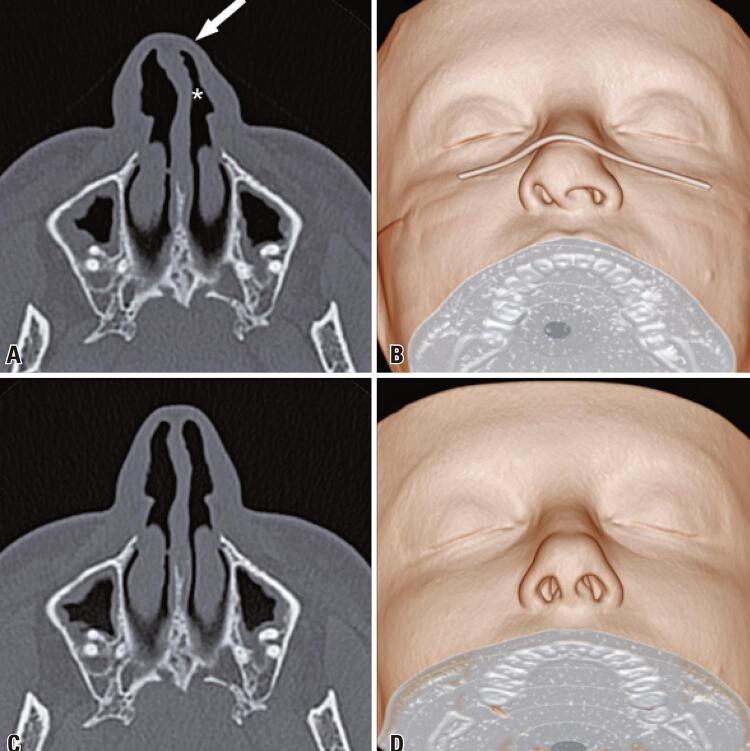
Computed tomography images of the same patient performed in 2021 (A and B) and 2019 (C and D). Axial (A) and three-dimensional volume-rendered (B) computed tomography images show significant deviation of the anterior cartilaginous portion of the nasal septum to the left, with a reduction of the left nasal vestibule (asterisk) and flattening of the nasal dorsum (arrow). These findings are associated with the use of a face mask. Axial (C) and three-dimensional volume-rendered (D) computed tomography images, performed two years before without the use of a face mask, show a slight sinuosity of the cartilaginous portion of the nasal septum; no significant reduction in the nasal air column is observed
